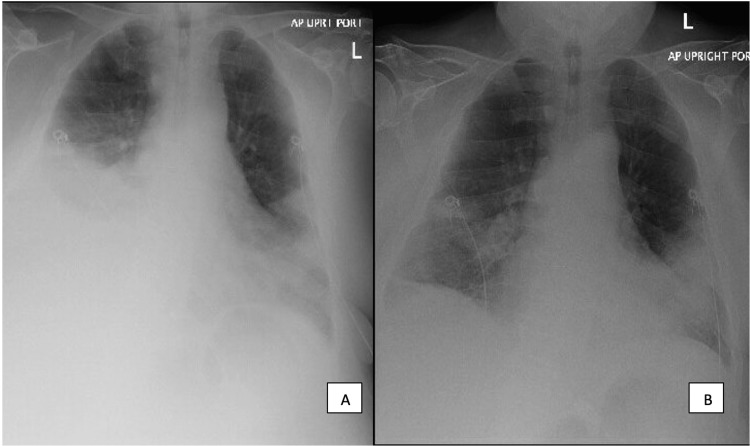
Chest radiograph after (A) left thoracentesis, (B) right thoracentesis.
